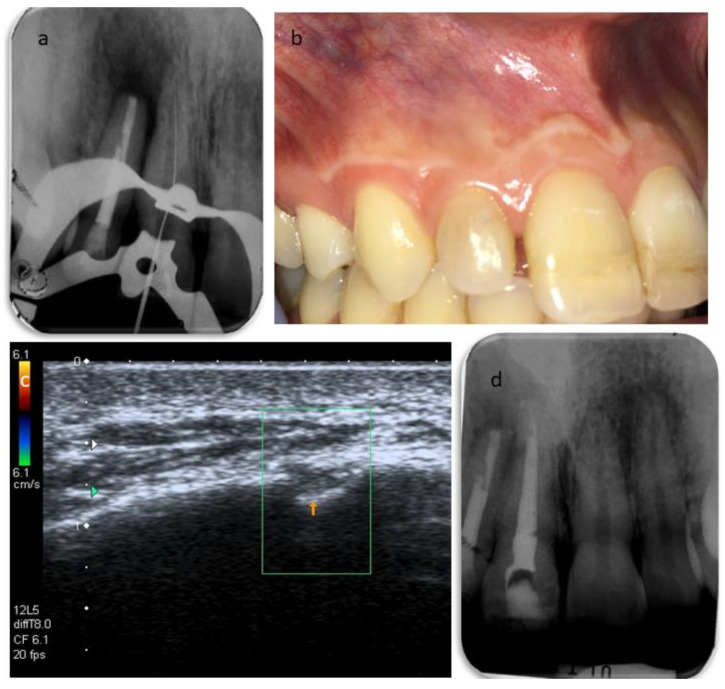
(a) Root canal treatment of tooth 1.1. (b) Clinical examination at one week after the root canal treatment, showing the disappearance of the stoma. (c) Ultrasound examination with the addition of the color Doppler showing the disappearance of the sinus tract and the vascular signal within the periapical lesion. (d) Post-operative periapical radiograph.
